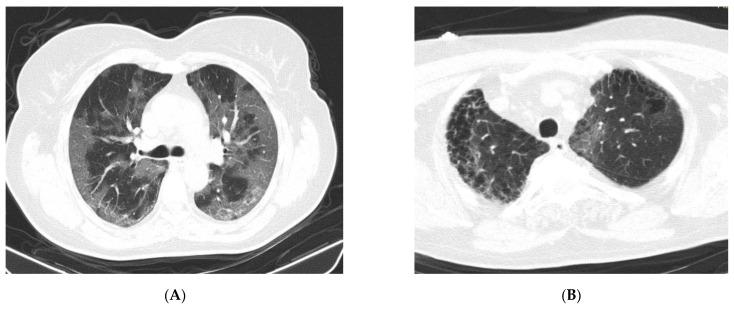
Native chest CT. (A) Images of extensive ground-glass opacity (GGO), distributed bilaterally, centrally, and peripherally. (B) GGO image in a left apical subpleural location (interstitial lesion in pulmonary fibrosis, with emphysema bubbles), suggestive of COVID-19 pneumonia (negative RT-PCR test) (from the collection of the radiology clinic, St. Spirid on Hospital, Iasi, Romania, May 2020).
